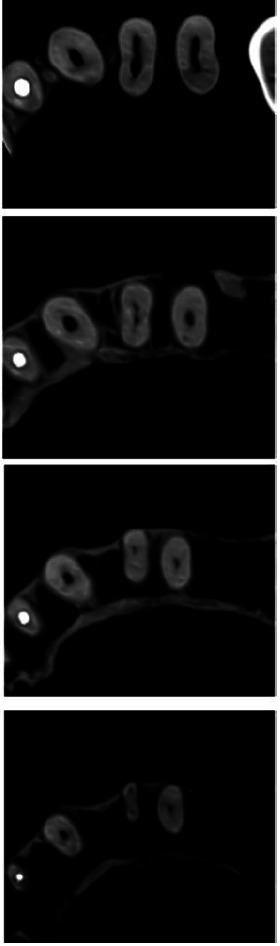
Cone beam computed tomography (CBCT) image of the tooth (axial view)
